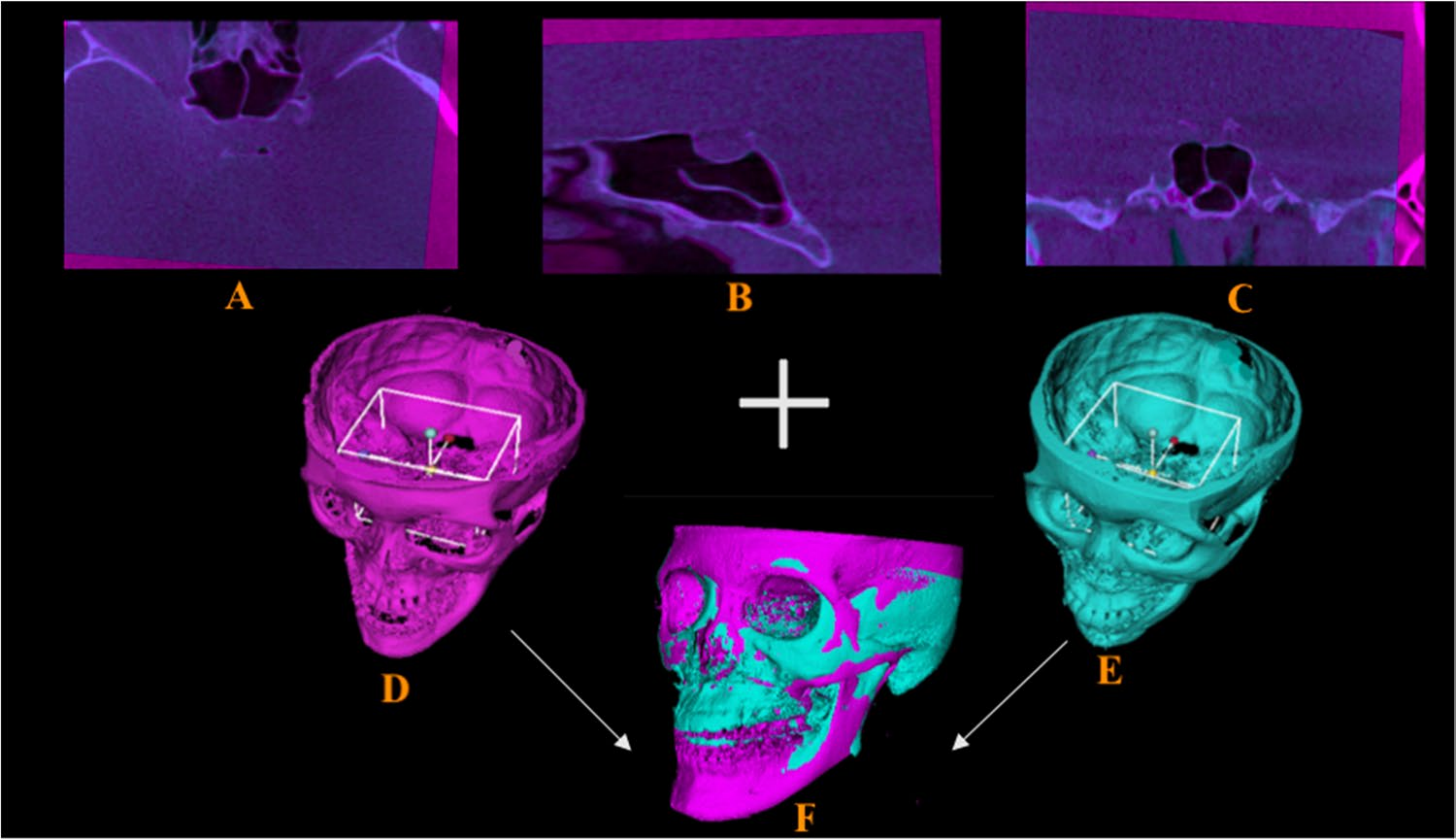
Representative images depicting the ROI for the registration of pre- and postoperative CBCT volumes based on the predefined stable structures of the cranium, where in pink signifies presurgery CBCT scan and blue signifies postsurgery CBCT scan. A, B, C ROI in axial, sagittal, and coronal views; D, E ROI in 3D reconstructed images (pre- and postsurgery respectively); F superimposed final 3D reconstructed image
